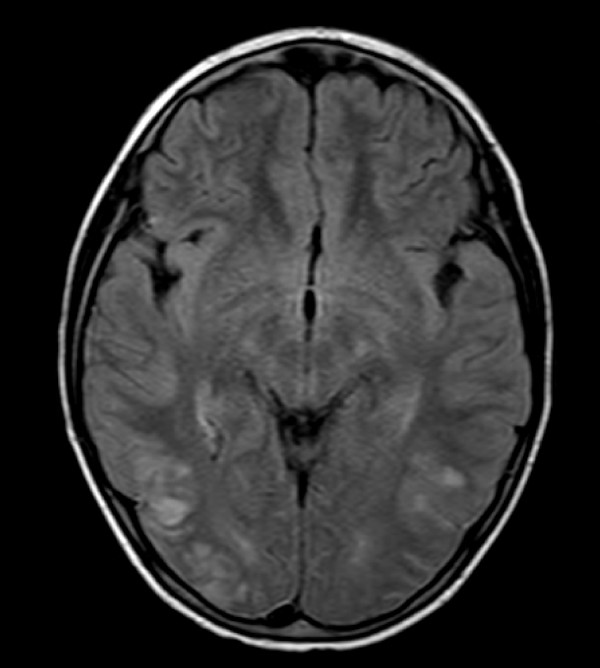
MRI-flair axial study of the brain (patient #3) showing multiple cortical and subcortical spots involving both occipital hemispheres.
